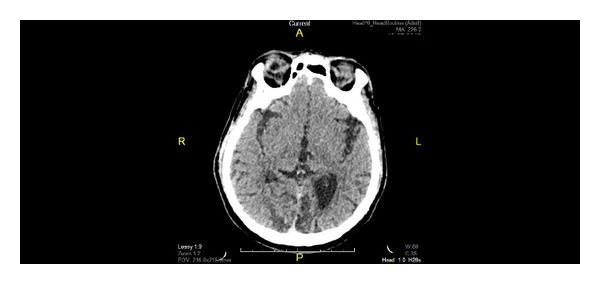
Noncontrasted CT brain shows bilateral occipital hypodense lesion with dilated left lateral ventricles.
